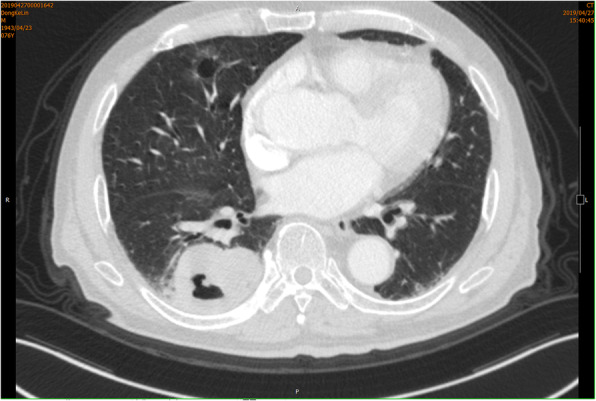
Chest computed tomography scan of the patient before operation. The right lung shows a 22 mm ground glass opacity with cavity in the middle lobe and a 61 mm solid mass with cavity and pleural indentation in lower lobe, with no hilar or mediastinal lymphadenopathy
